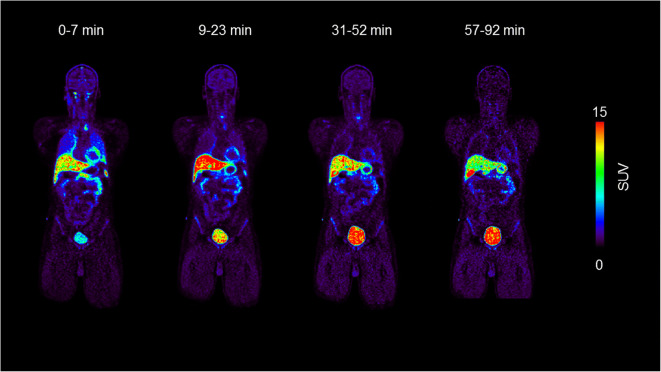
Coronal whole-body views showing biodistribution in one representative male subject (34 years, 92 kg) at different time points after injection of 353 MBq [^11^C]metoclopramide. Radiation scale is given in SUV units and set from 0 to 15.
